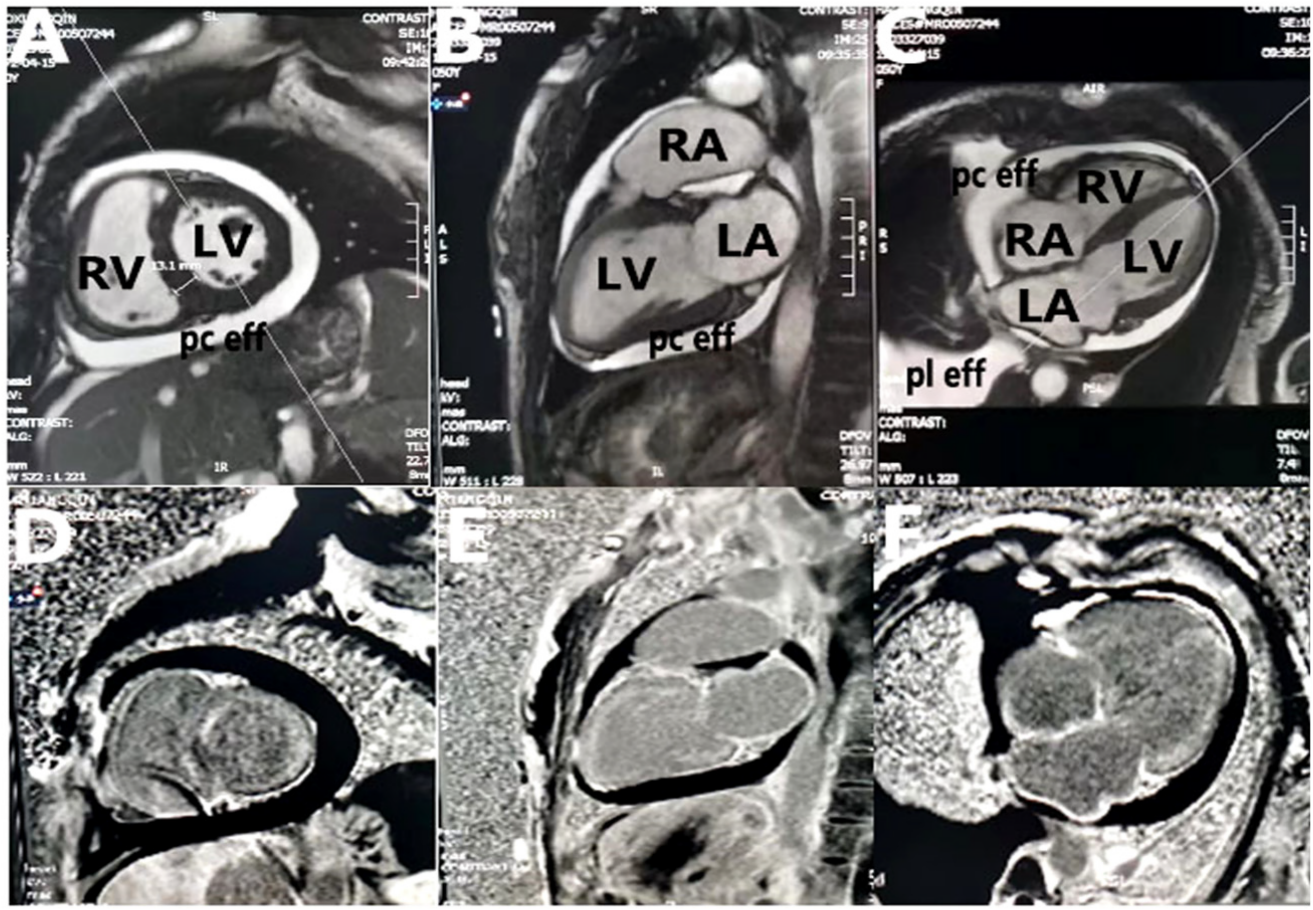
Cardiac magnetic resonance imaging. (A–C) Two-chamber, three-chamber, and four-chamber steady-state free precession images demonstrate mild biatrial enlargement, increased left ventricular wall thickness (13 mm), pleural effusion, and pericardial effusion; (D–F) two-chamber, three-chamber, and four-chamber views from postgadolinium delayed enhancement images show left atrium, right atrium, right ventricle, and left ventricle line-like enhancement. LA, left atrium; RA, right atrium; LV, left ventricle; RV, right ventricle; pc eff, pericardial effusion; pl eff, pleural effusion.
